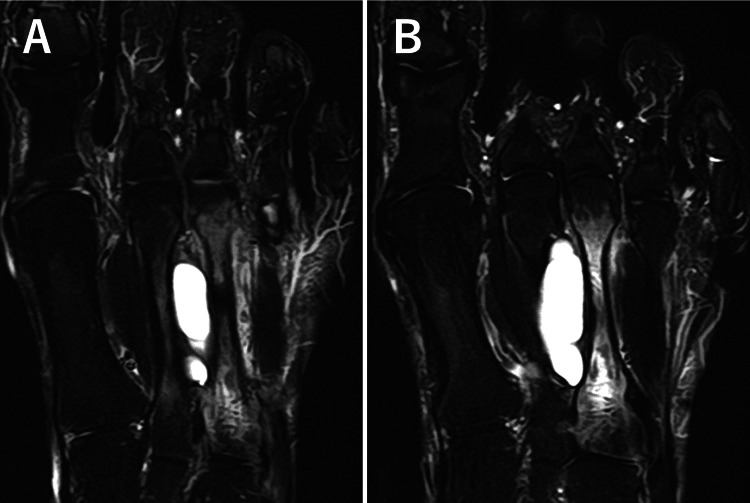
MRI of the right foot. T2-weighted image showing a high-intensity cystic mass lesion between the second and third metatarsals with high signal intensity in both metatarsals (A and B).
